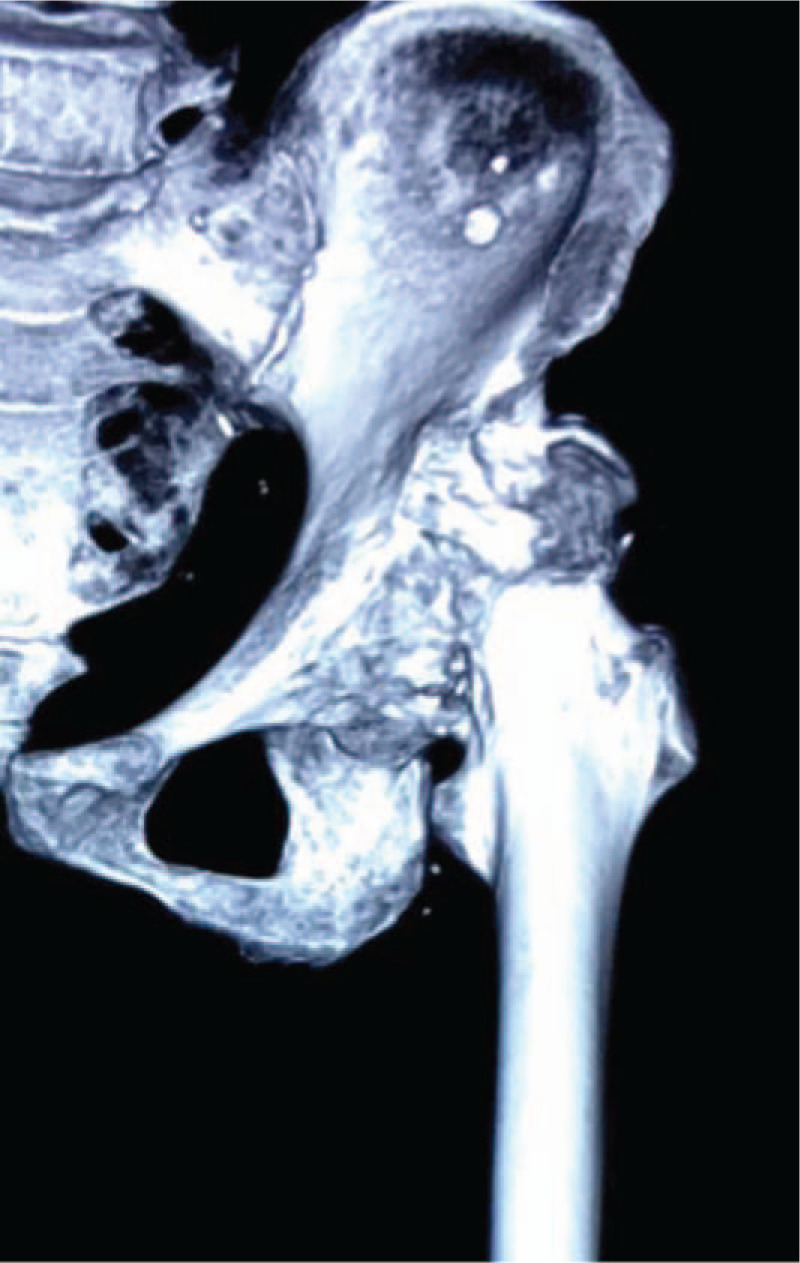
Preoperative 3-dimensional-computed tomography. 3D = 3-dimensional.
